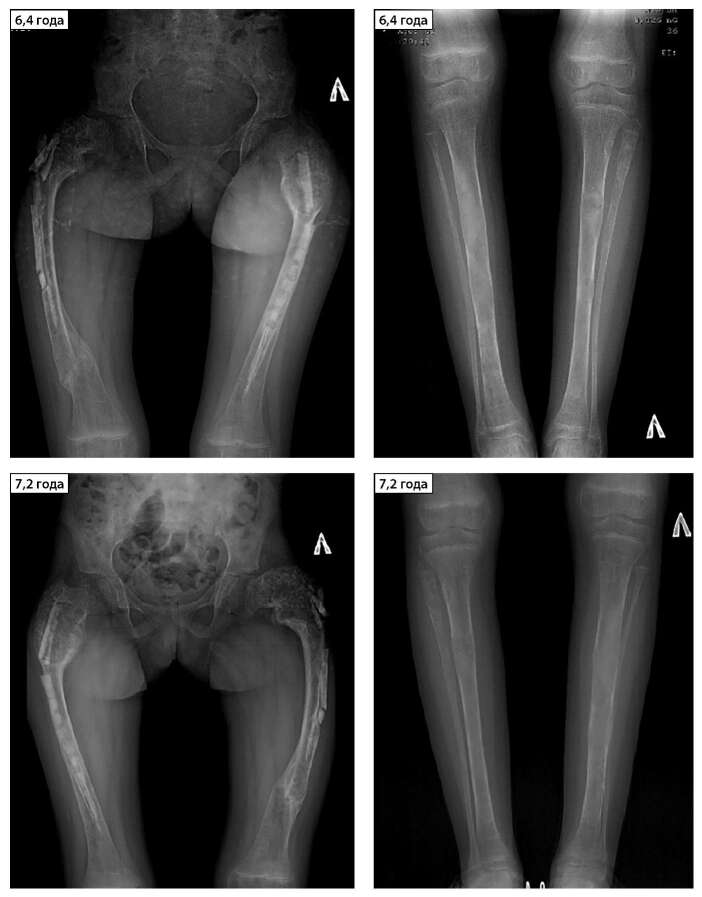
Рисунок 1. Рентгенограммы и компьютерные томограммы нижних конечностей и позвоночника в динамике.Патологические переломы в течение жизни: правой бедренной кости в 4 года, основной фаланги 2 пальца правой стопы в 5,2 года, правой плечевой кости в 5,6 года; правой большеберцовой кости в 5,6 года (через 1 мес после предыдущего); левой бедренной кости в 6 лет. Ортопедические хирургические лечения в течение жизни: внутриочаговая резекция очагов фиброзной дисплазии, металлоостеосинтез левой бедренной кости в 3,6 лет; металлоостеосинтез правой бедренной кости в 4,5 года; удаление металлофиксаторов левой бедренной кости в 5,8 лет (нестабильность металлоконструкции); остеотомия и остеосинтез левой бедренной кости в 8,6 года. Рентгенограммы нижних конечностей в 6,8–9,7 года: прогрессирующая деформация бедренных костей по типу пастушьего посоха, тотальная фиброзная перестройка костной ткани бедренных костей с визуализирующимися металлоконструкциями; очаги фиброзной дисплазии в костях голени с прогрессией в тотальную фиброзную перестройку костной ткани и с формированием саблевидной деформации костей левой голени к 9,2 года. Рентгенограммы позвоночника в 7,8 года: кифосколиоз без очагов фиброзной дисплазии.Figure 1. X-ray and computed tomography of the lower extremities and spine in dynamics.
